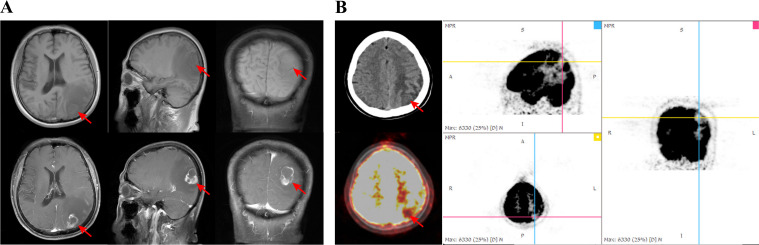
Solitary brain metastasis. (A) Non-contrast and contrast-enhanced T1-weighted MRI in the axial, coronal and sagittal planes showed a focal solid tumor in the left parieto-occipital lobe (arrow). (B) PET/CT showing evidently reduced radiosensitivity centrally and increased uptake in the peripheral ring (arrow).
